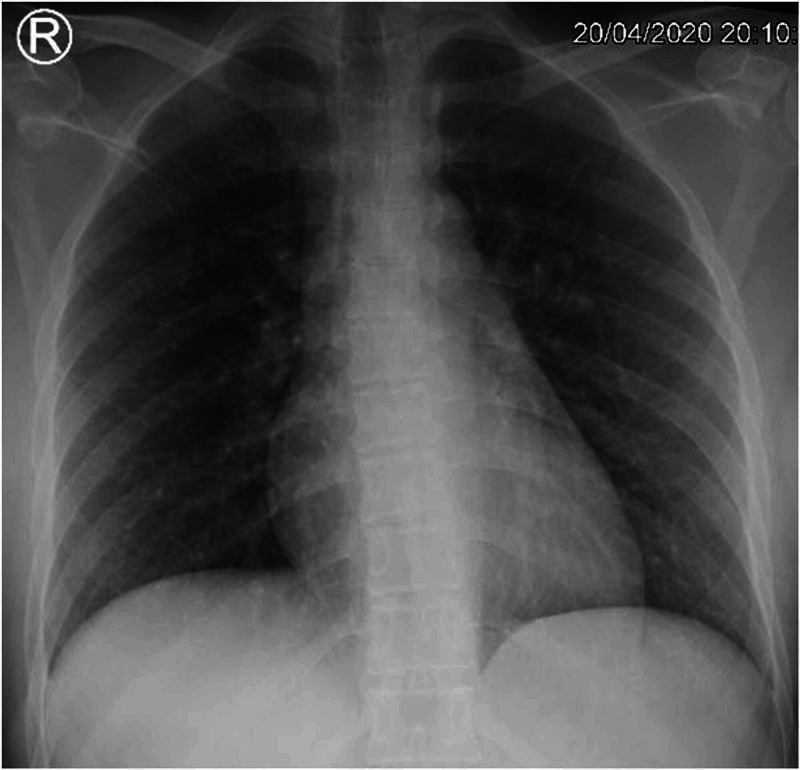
Chest X-ray upon admission for case 1.
